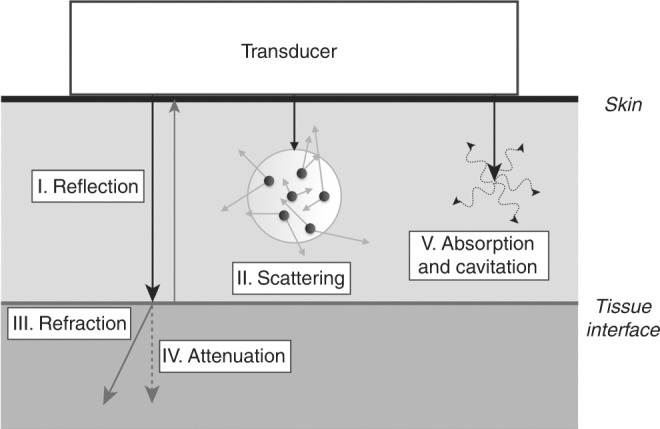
Ultrasound and tissue interactions. Adapted from Textbook of Clinical Echocardiography, 5th Edition, Catherine Otto, “Principles of Echocardiographic Image Acquisition and Doppler Analysis,” Fig. 1.4, page 5, Copyright (2013), with permission from Elsevier
